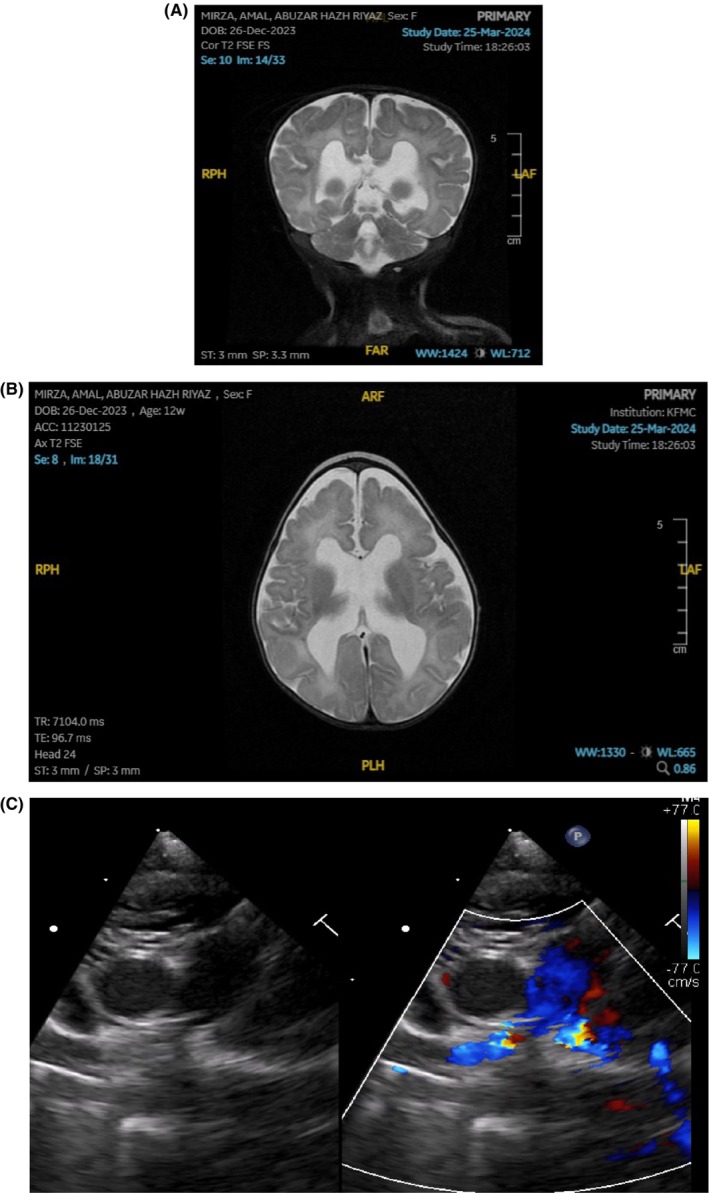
(A) Coronal view of T2 brain MRI. (B) Axial view of T2 brain MRI. (C) Echocardiographic image of the heart dynamics following insertion of right pulmonary artery flow regulator.
